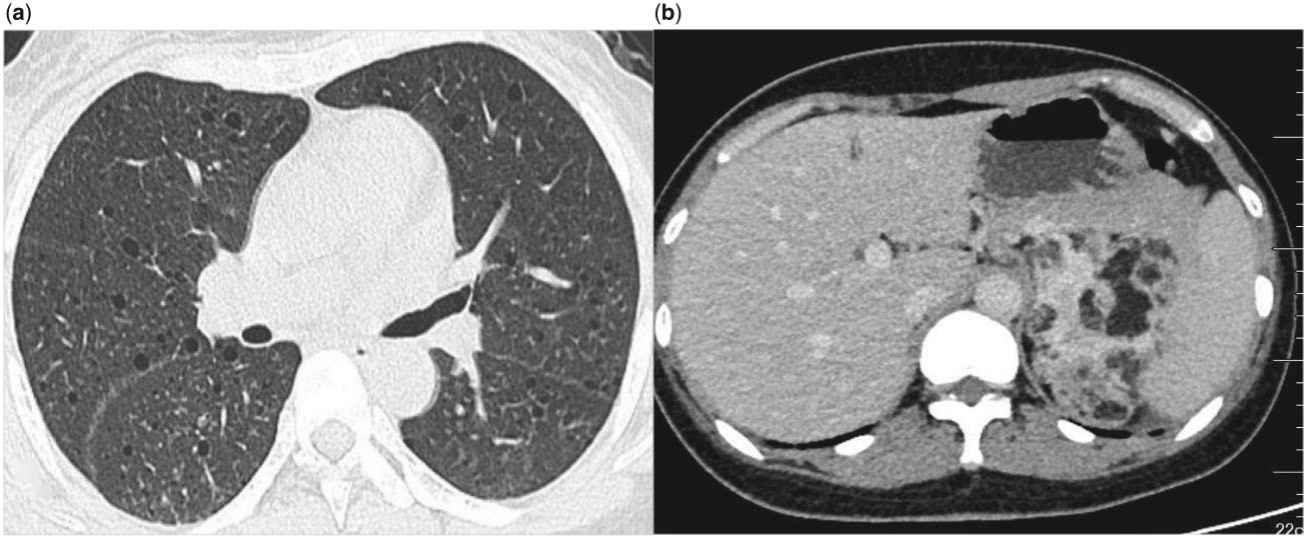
(a) Chest CT scans showing multiple thin-walled, round, well-defined cysts and nodules of variable sizes bilaterally. (b) Abdominal CT showing multiple hamartomas of the liver and left kidney.
